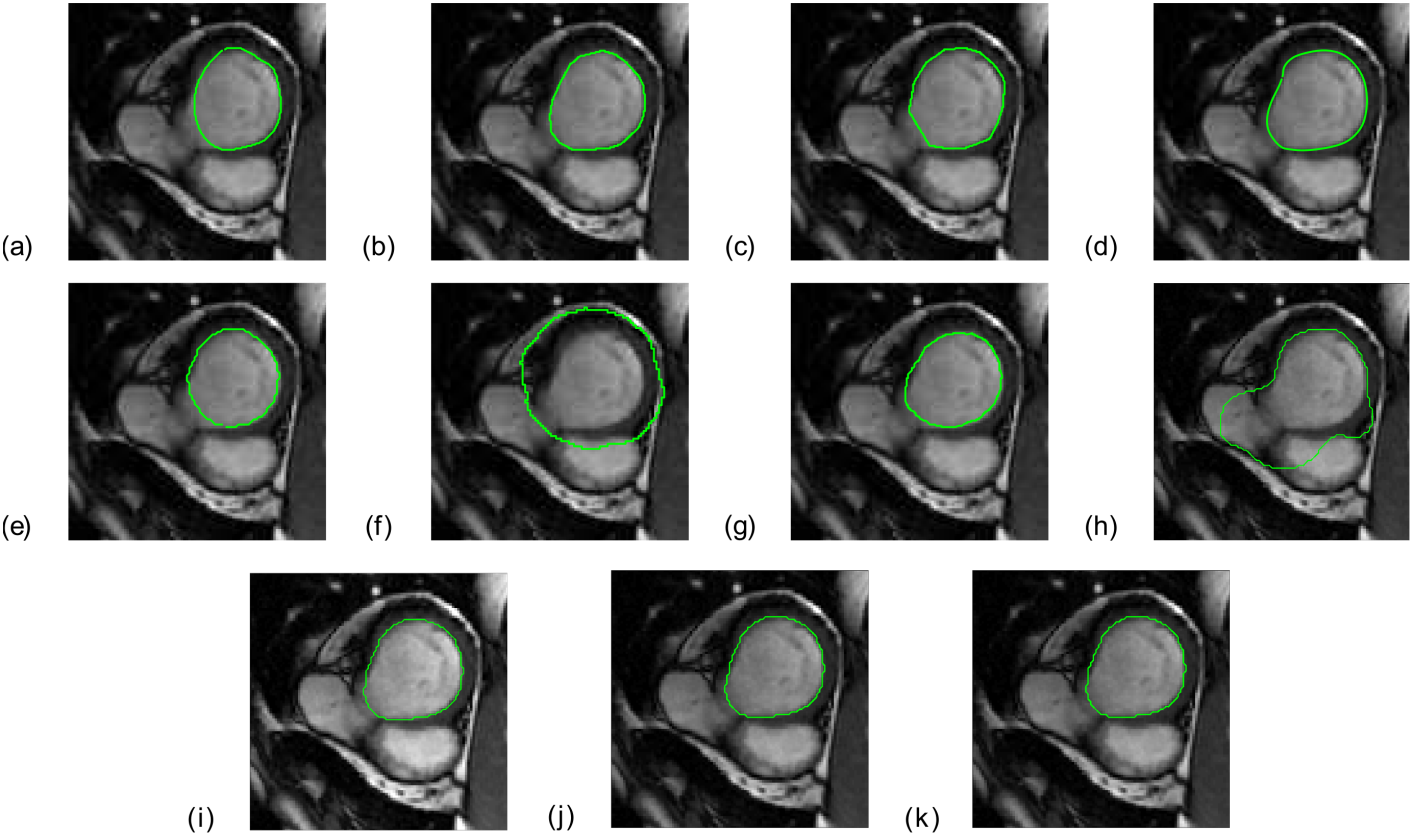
Basal cine MRI slice at end-diastole with superimposed contours of the LV (green line). *M*1 to *M*8 are represented from (a) to (h) and three different combinations of the STAPLE algorithm, *MS*45678, *MS*456 and *MS*4578 are represented from (i) to (k).
